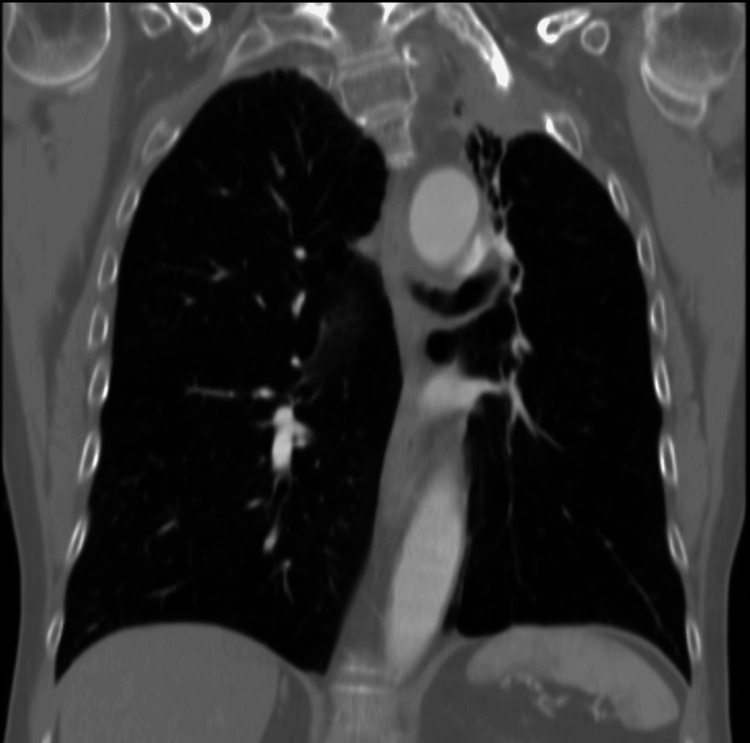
Coronal CT scan with IV contrast of the lungs. There are fibrotic changes, particularly in the left apical lung, with slight thickening of the more central airways on the left
